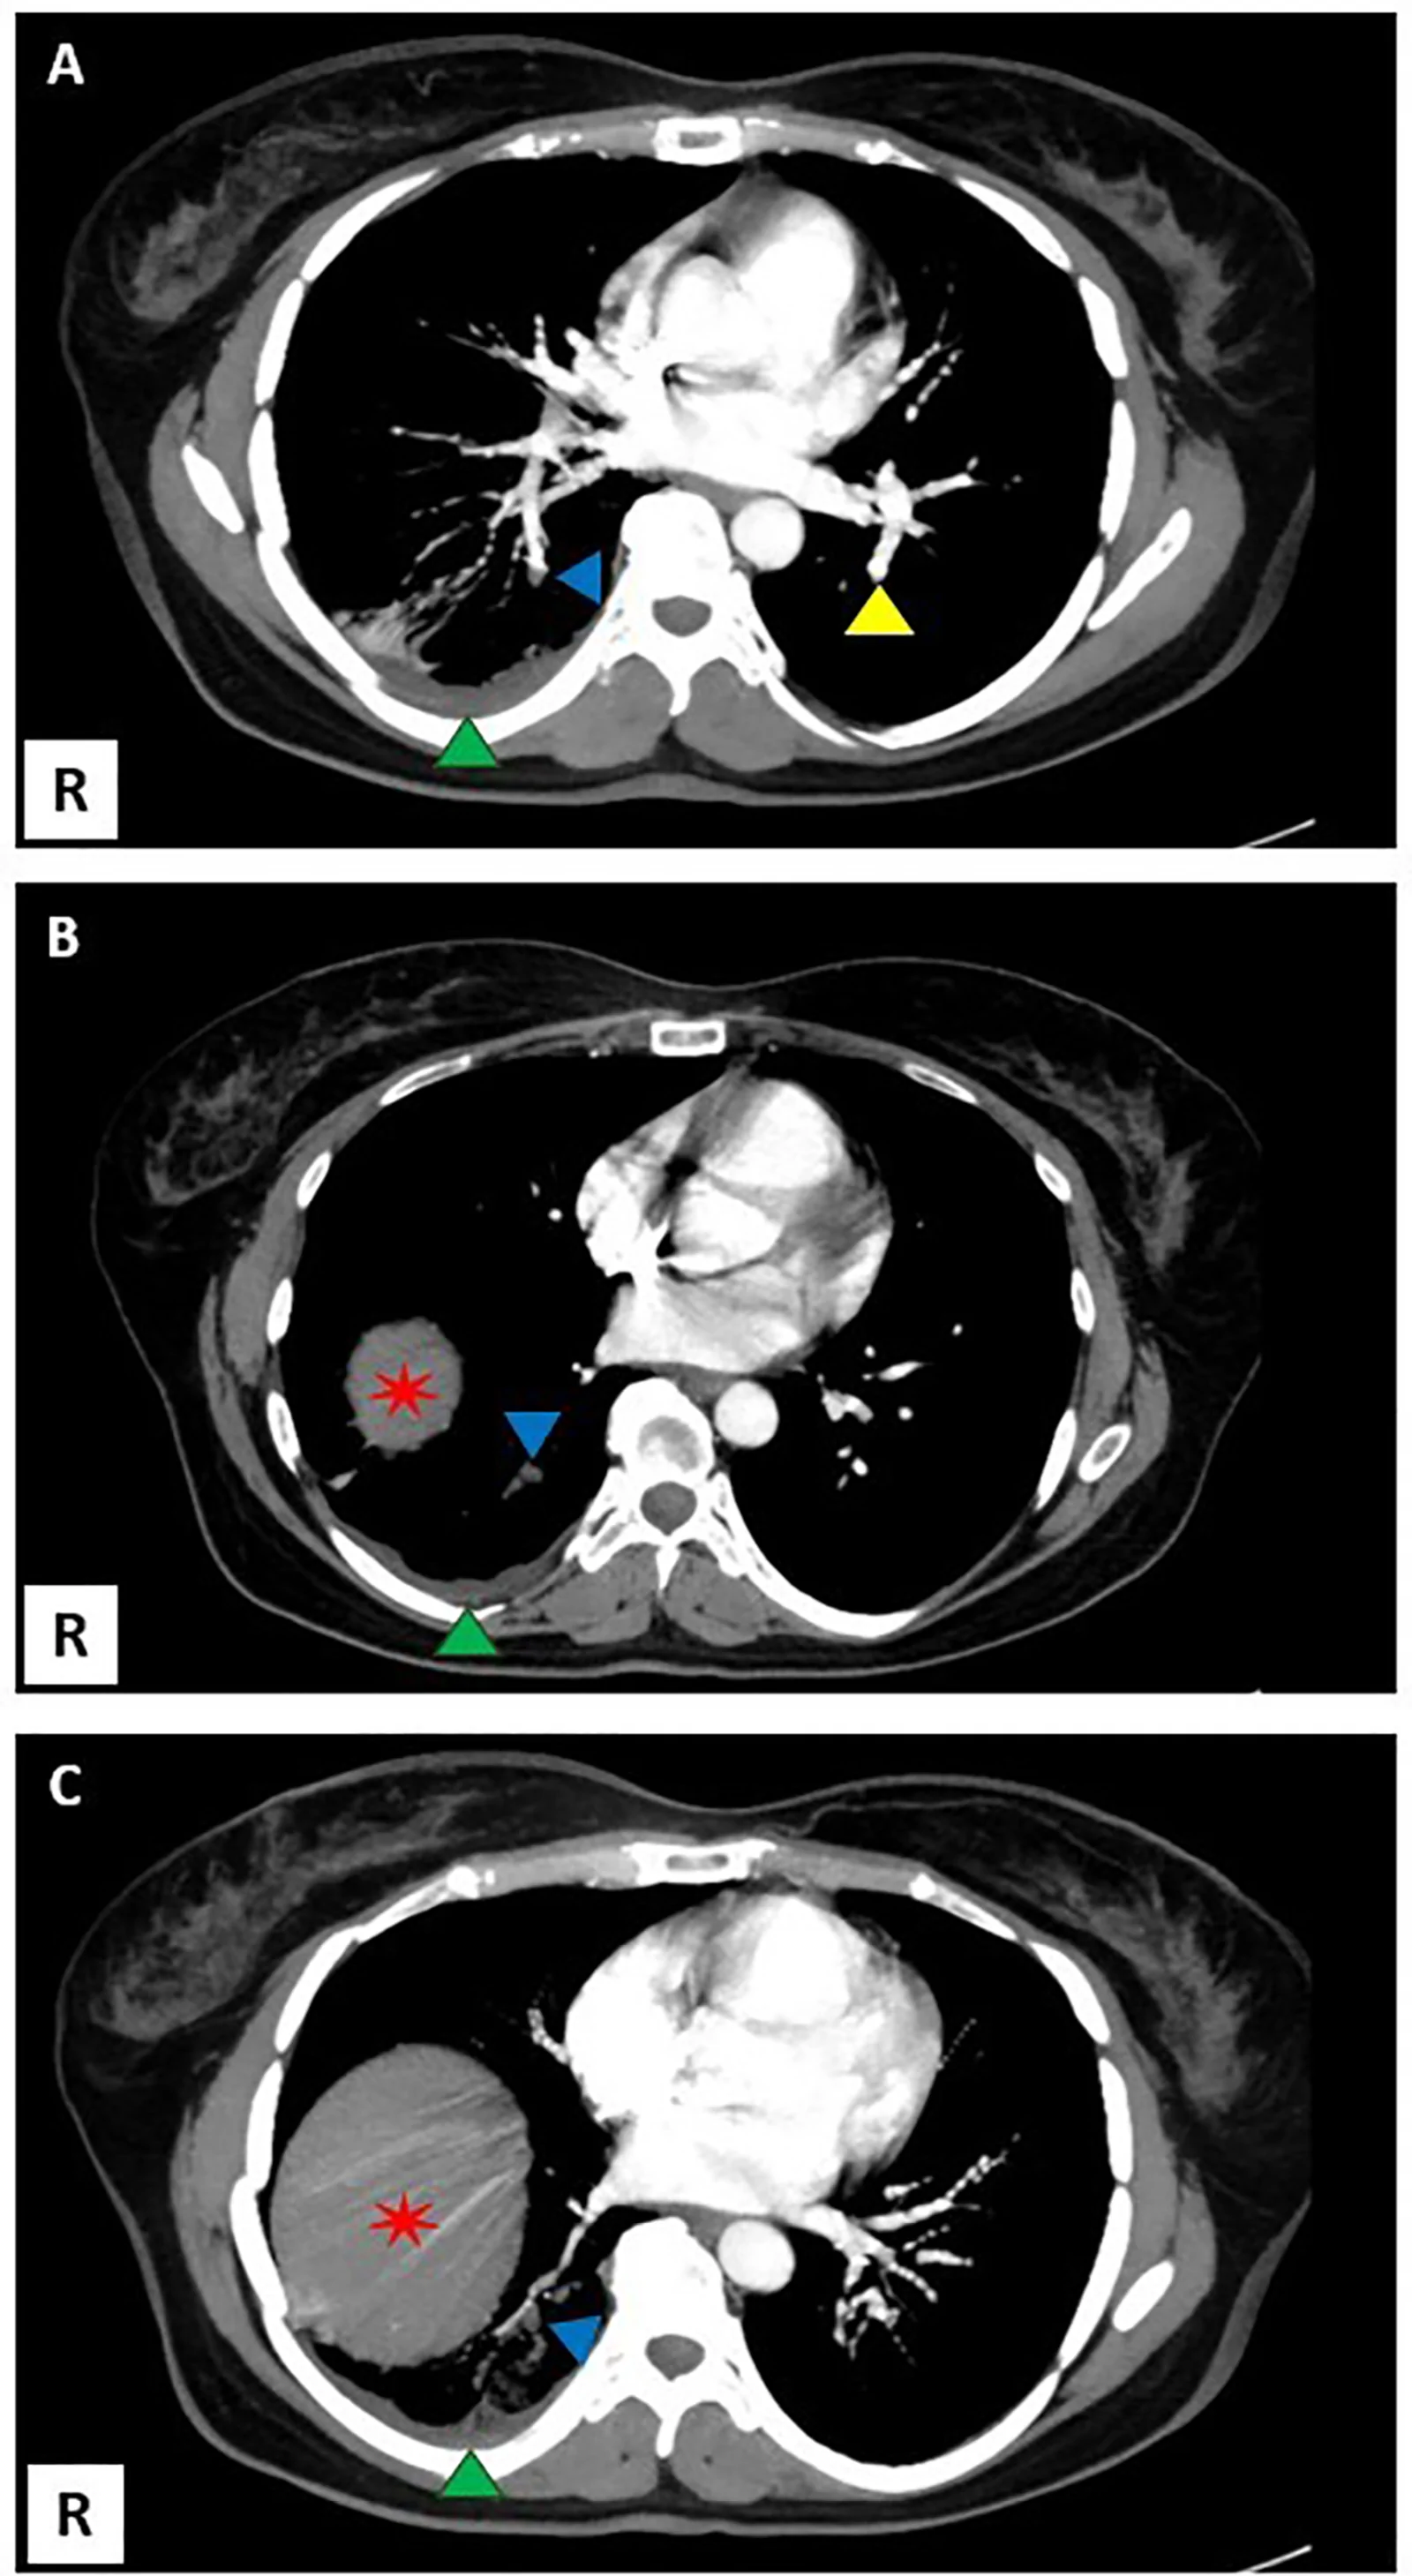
CT angiography at multiple levels, showing 3 sequential slices (from A to C). In A, there is an occlusive pulmonary embolus represented by a filling defect at the right subsegmental branch at which the contrast stopped (blue head arrow). The cutoff flow of the contrast in the vessel is indicated by a hypodense spot (the embolus) versus the normal cut on the left side marked by the yellow head arrow. The green head arrow indicates pleural effusion. The red asterisk indicates an elevated diaphragm, consistent with a collapsed right lung base. R indicates the right side.
